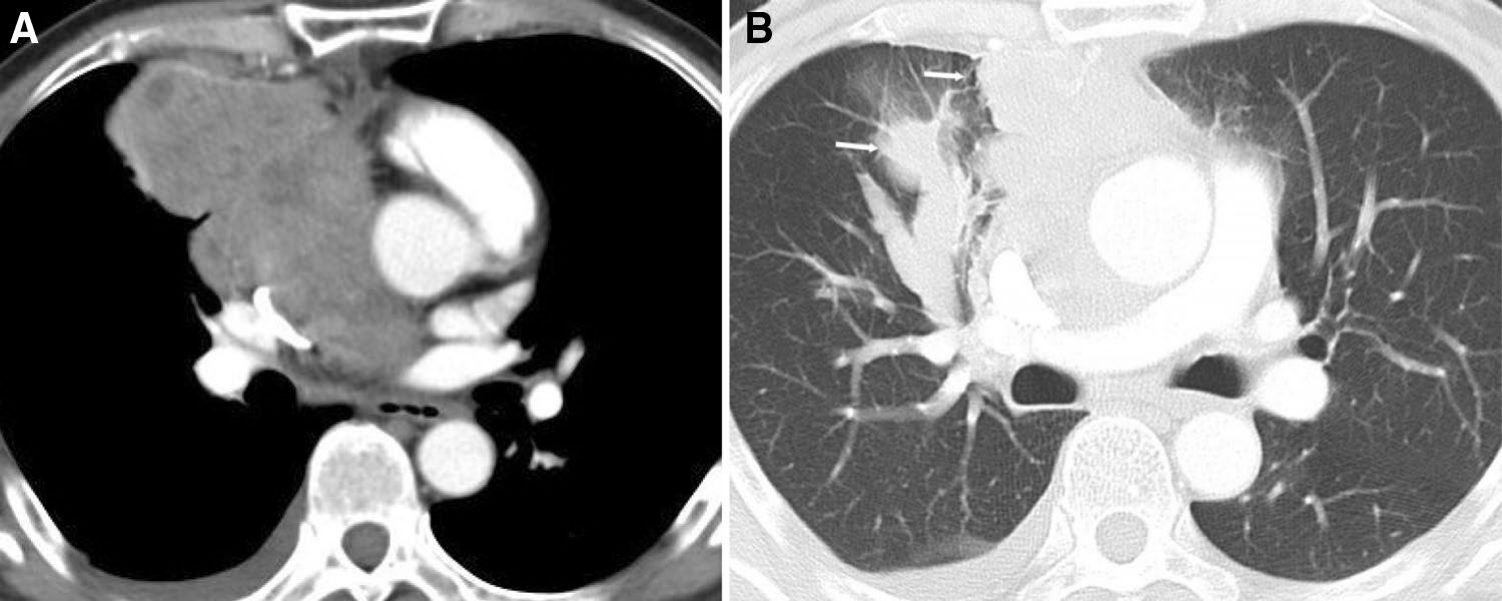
High-risk thymoma (type B2) in a 66-year-old man with pericardial mass and lung invasion. Contrast enhanced CT scan (Axial 5.0 mm) obtained at the level of the aortic root (A) and pulmonary trunk (B) shows tumor invading the pericardium and form large soft tissue mass inside the pericardium. The interface between the tumor and lung was lost, with compression and effusion in the lung (white arrow). This patient died 23.8 months since the first CT examination.
